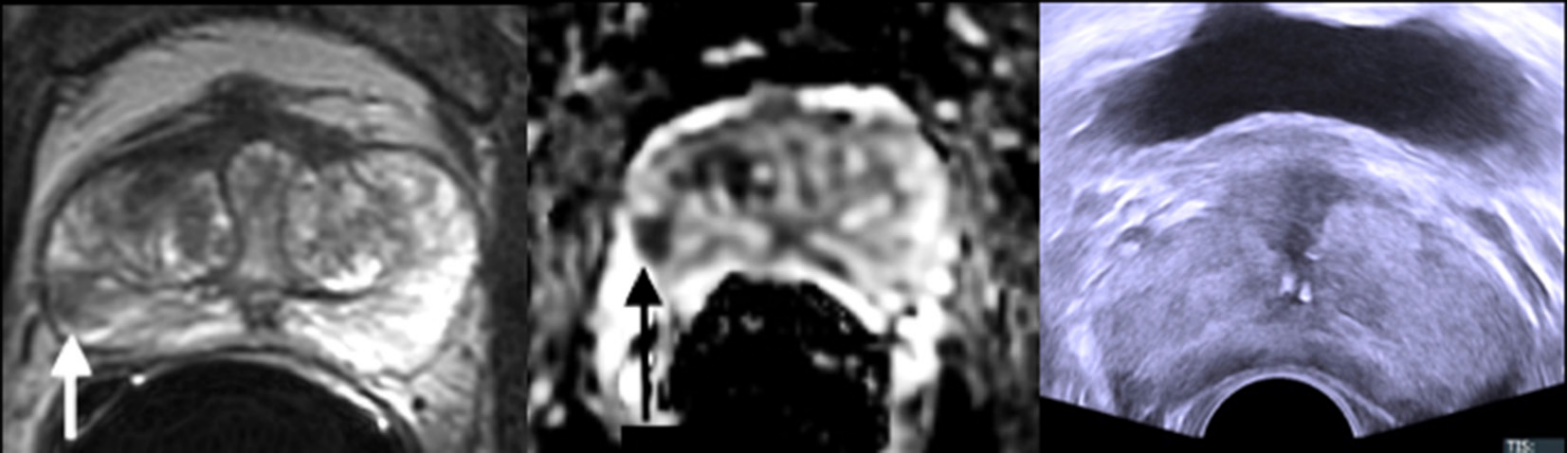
A lesion in the right mid peripheral zone demonstrates T2 hypointensity (a) and a very low ADC (b), compatible with a PI-RADS score of 4. Nevertheless, TRUS in the same patient demonstrates no clear hypoechoic correlate (c), leaving the operator to estimate the target position on the ultrasound image, which may be difficult in a homogeneous gland. This target was shown to represent GS 4 + 4 cancer on prostatectomy. ADC,apparent diffusion co-efficient; AS, active surveillance; GS, Gleason score; TRUS,transrectal ultrasound.
